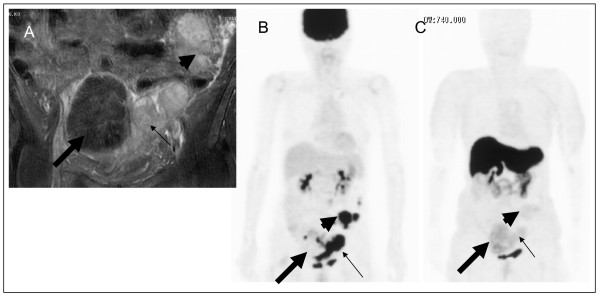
A 66-year-old woman with a diagnosis of ovarian cancer and huge uterine leiomyoma underwent PET. MRI demonstrated a huge uterine leiomyoma (large arrow) and left ovarian cancer (small arrow) with metastases in the abdomen (arrow head) (A). FDG-PET demonstrated ovarian cancer (small arrow) and multiple metastases in the abdomen and pelvis (arrow head), and a negative FDG-PET scan is shown for the leiomyoma (large arrow) (B). FES-PET demonstrated moderate uptake of FES in both the ovarian cancer (arrow head) and its metastases (arrow head) and leiomyoma (large arrow) (C).
